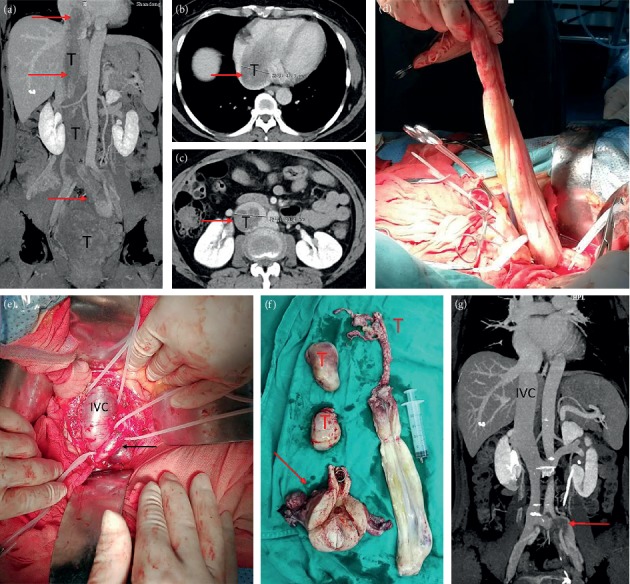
Case 3. (a, b, c) Tumor entered the right atrium from the right iliac vein through the inferior vena cava, and the tumor diameter in the right atrium was 4 cm. (d) The tumor was pulled out from the inferior vena cava. (e) The arrow shows the left common iliac vein compressed by the right common iliac artery. (f) The arrow points to the resected uterus and bilateral adnexa. (g) Postoperative CT shows unobstructed right atrium and inferior vena cava, and the arrow indicates left common iliac vein thrombosis.
